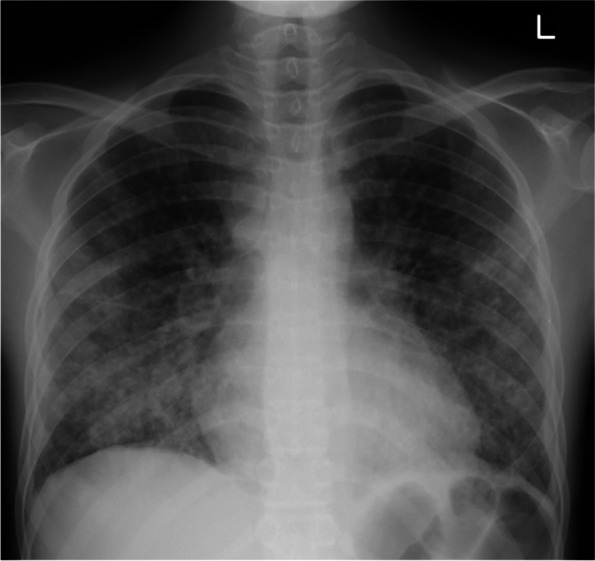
Chest radiograph showing lungs with bilateral miliary nodules
